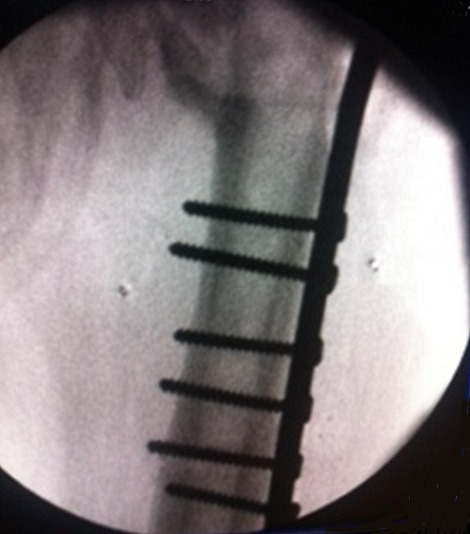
Intra-operative antero-posterior radiograph of the revision fixation
